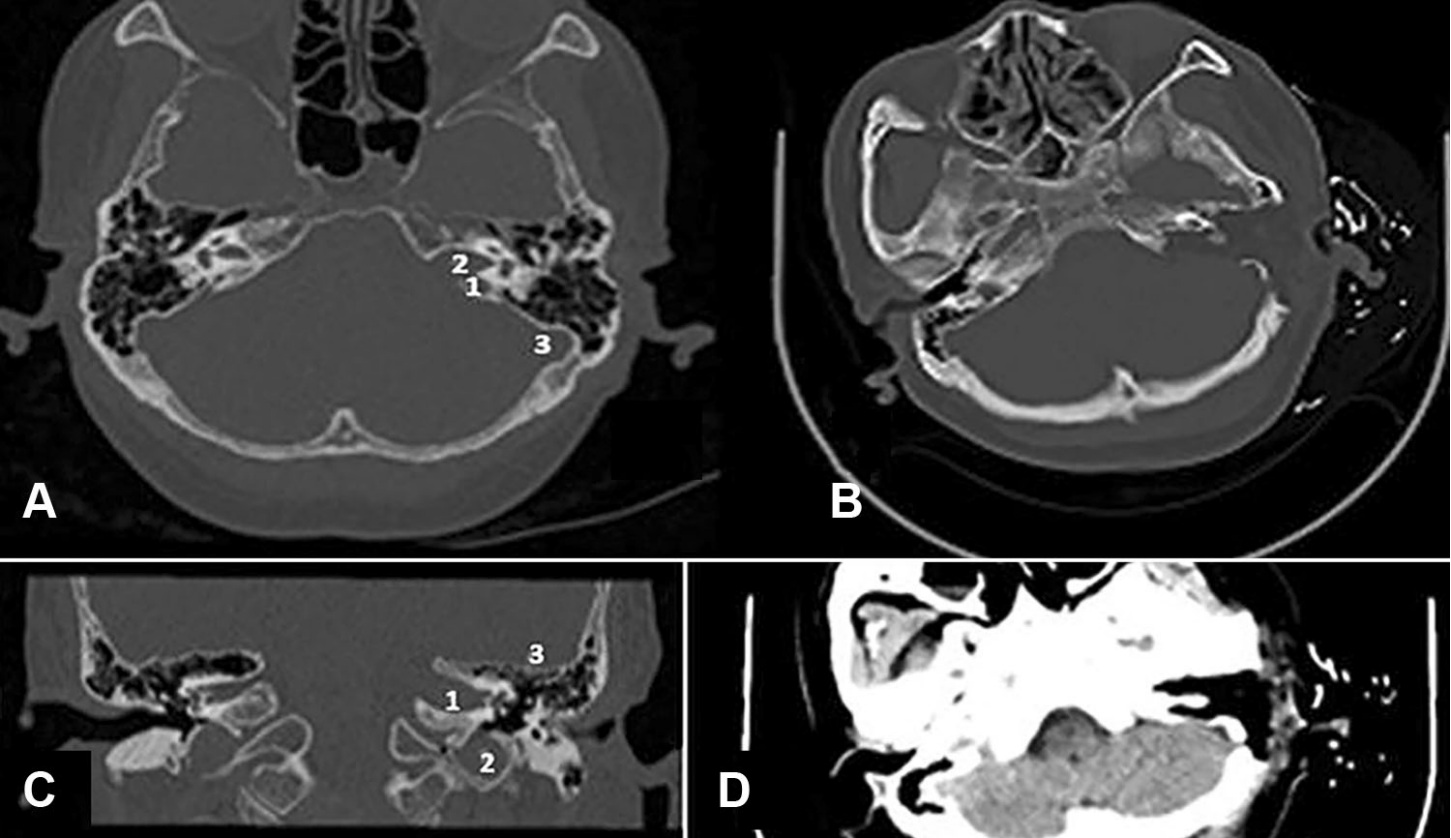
a The axial CT shows the high jugular bulb, the enlarged internal auditory canal with the acoustic tumor. *1* high jugular bulb, *2* enlarged internal auditory canal, *3* sigmoid sinus, normal middle cranial fossa dura and pneumatization. b The postoperative axial CT demonstrates the obliterated subtotal petrosectomy with radical tumor removal. c The coronal HRCT shows the left IAC enlarged by a vestibular schwannoma. *1* enlarged internal auditory canal, *2* high jugular bulb, *3* normal middle cranial fossa dura and pneumatization. d The postoperative axial CT of the same patients showing the operative cavity with the radical tumor removalMRI scans of the internal auditory canal cerebellopontine angle and posterior cranial fossa were obtained in all 36 patients via GE Signa 1.5T MRI scanner (T1 weighted post-gadolinium sequences), to determine tumor size. The tumors were 0.5–2 cm in 13 patients (36%), 2–4 cm in 18 (50%) patients and 4–5 cm in 5 patients (14%).
